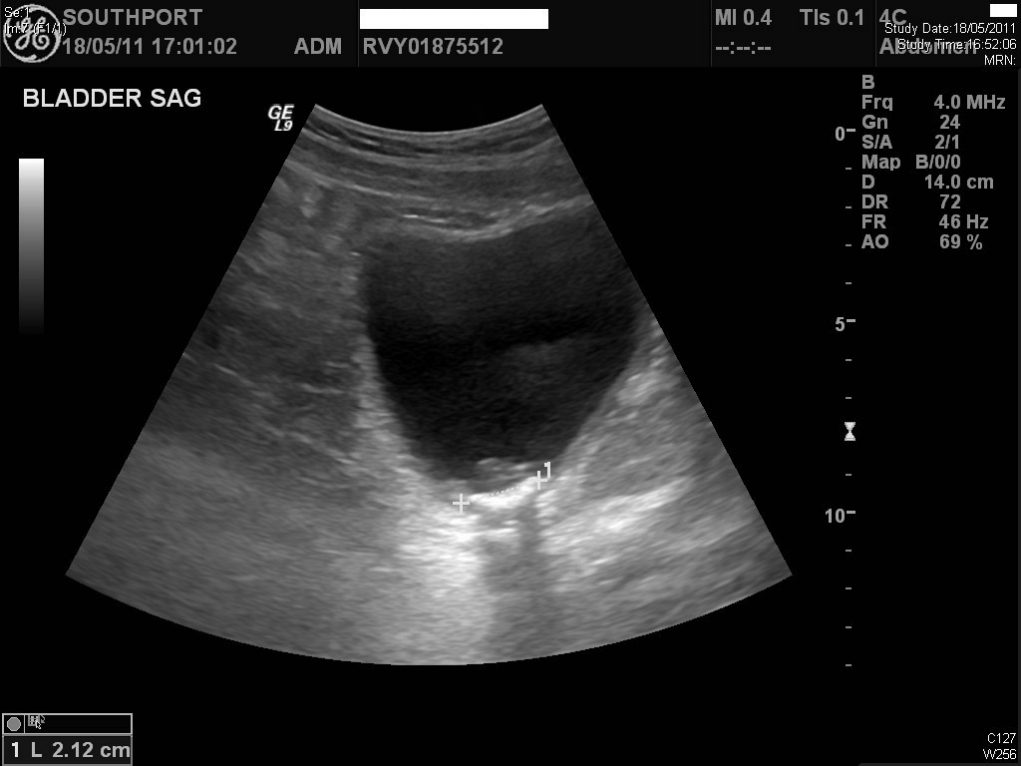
Ultrasound examination of urinary bladder, performed on 18 May 2011, revealed multiple calculi seen posteriorly within the bladder, the largest one measuring up to 2 cm. This patient did not develop autonomic dysreflexia, when lithotripsy was performed under subarachnoid block.
